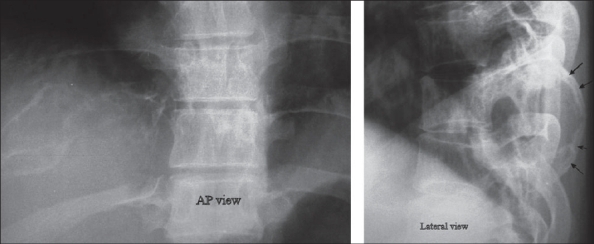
Preoperative radiographs showing cyst in the eighth rib. Notice that the normal outline of the right-sided eighth rib is not visible due to the expansile lytic lesion. Lateral X-ray shows the expansile lytic lesion (arrows)
